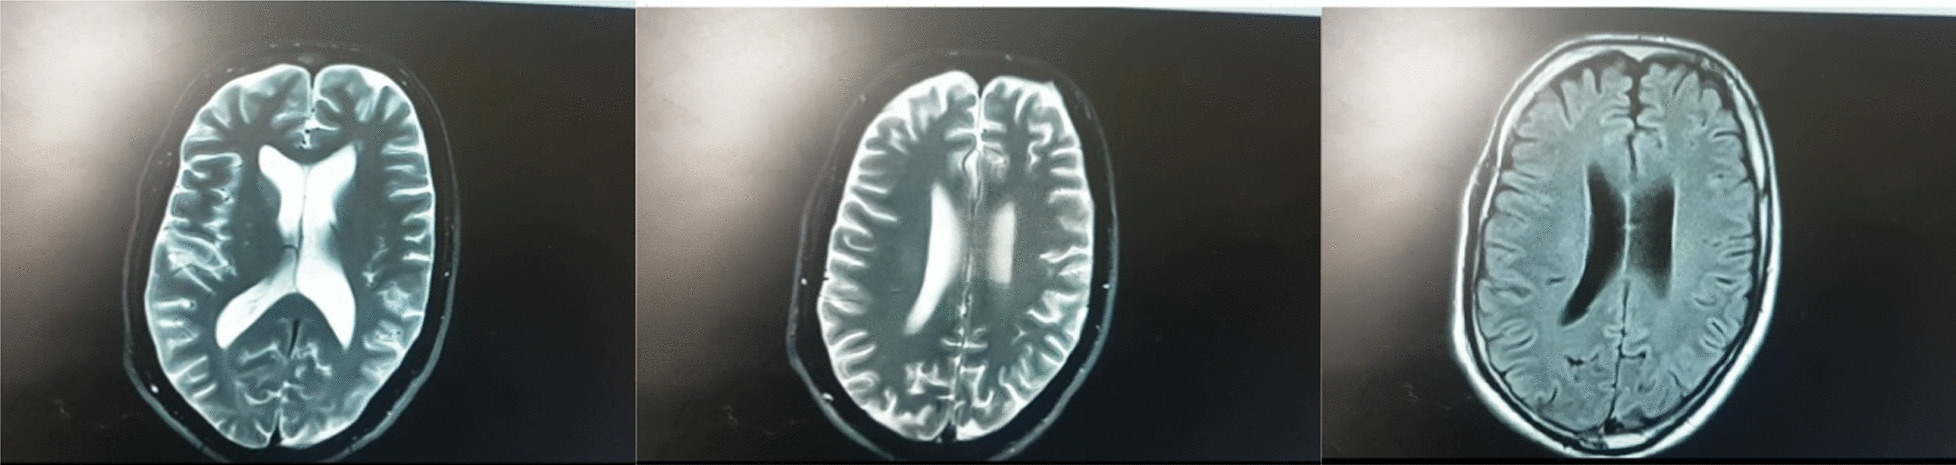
Brain magnetic resonance imaging with diffusion-weighted imaging
